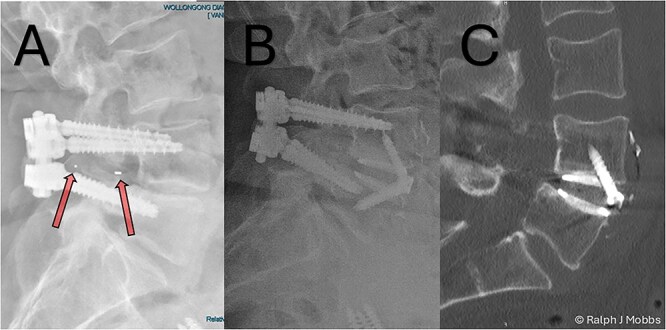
(A) Initial presentation with TLIF cage migration posteriorly into the spinal canal and paraspinal muscles. (B) Stage 1 and 2 completion, including revision fusion using an ALIF cage (Redmond Ti/PEEK, A-spine, Taiwan) and TLIF cage removal. (C) CT scan post TLIF removal and disc height restoration with ALIF cage.
